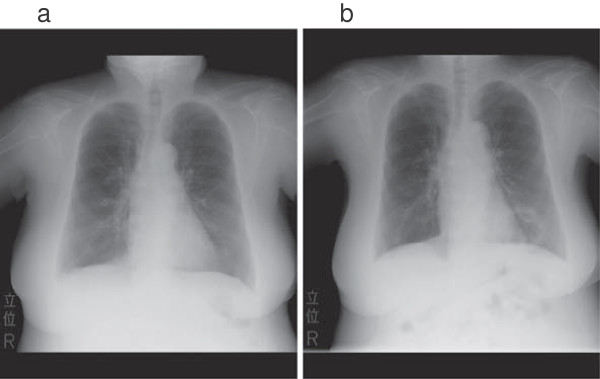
Chest radiographs obtained one month before admission (a), and on admission (b). A large hollow lesion in the lower left lung field was diagnosed as a lung abscess.
